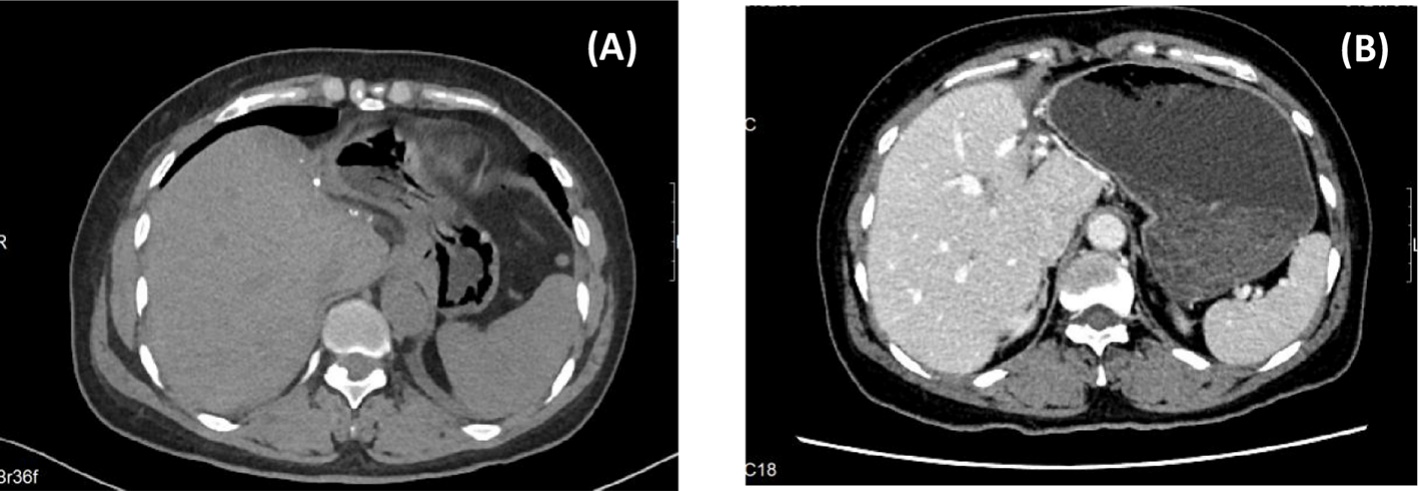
Postoperative FOLLOW-UP. [(A) CT images at 1 month after surgery; (B) CT images at 6 months after surgery.].
